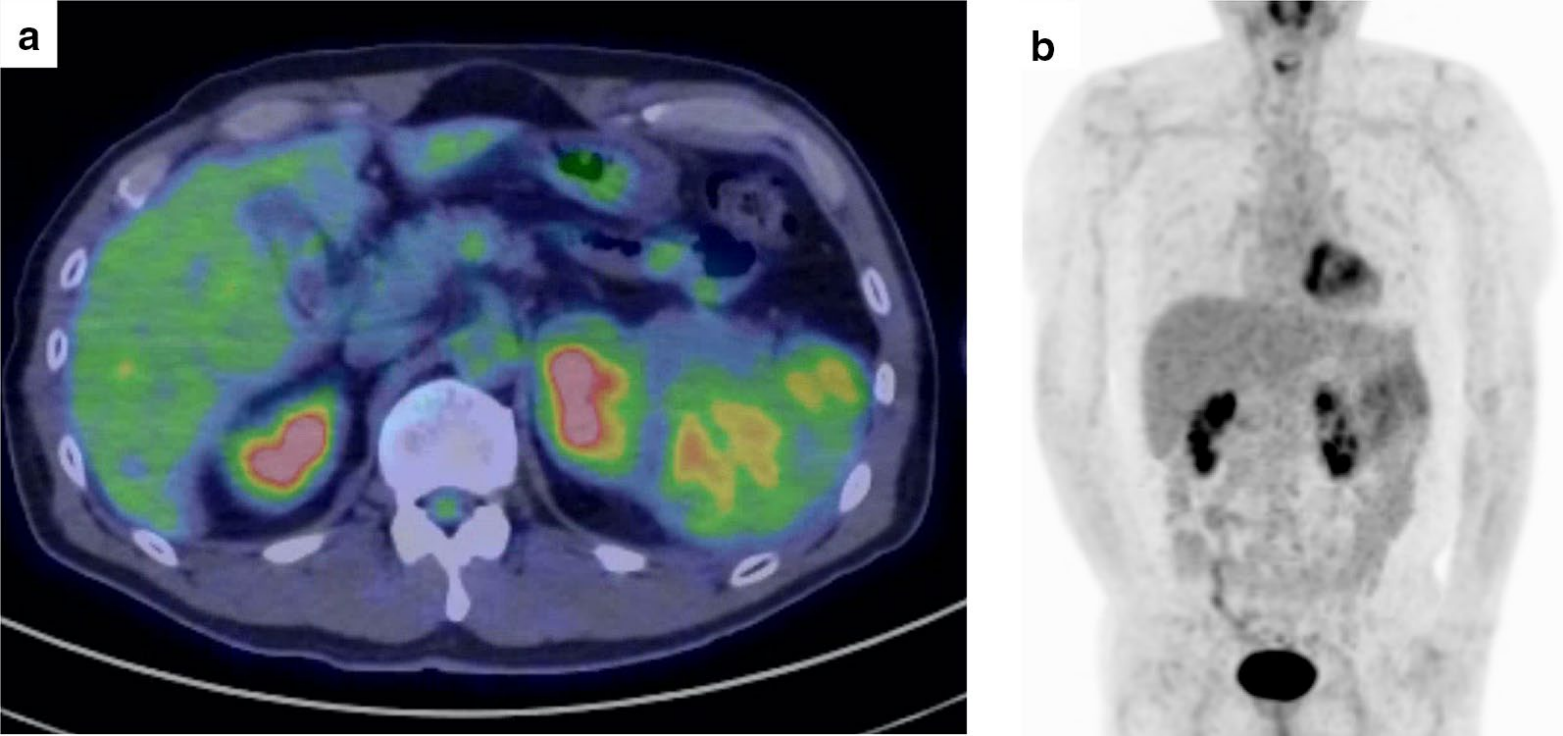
a PET–CT showed an accumulation of standardized uptake value (SUV) max of 4.1. b PET–CT showed no obvious lymph node or distant metastasis
